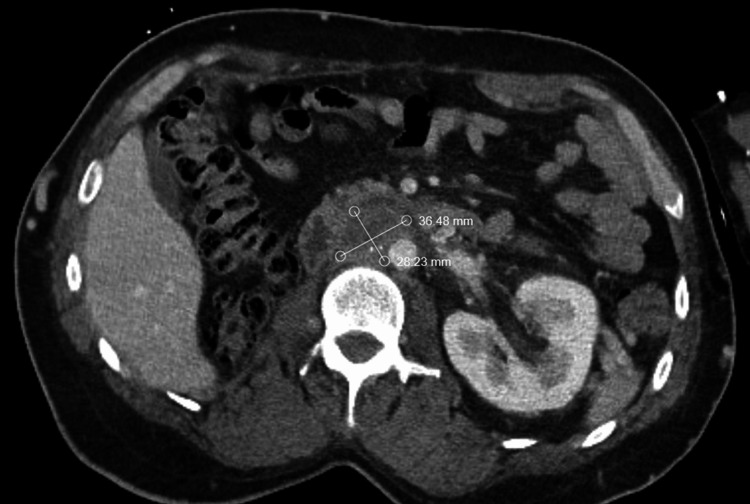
Latest surveillance axial computed tomography (CT) images of the abdomen and pelvis Axial CT imaging scans with IV contrast re-demonstrating retroperitoneal lymph nodes (B) 15 months after completion of radiation therapy. Measurements of residual disease demonstrate no significant progression by RECIST v1.1 criteria. RECIST: Response Evaluation Criteria in Solid Tumours
